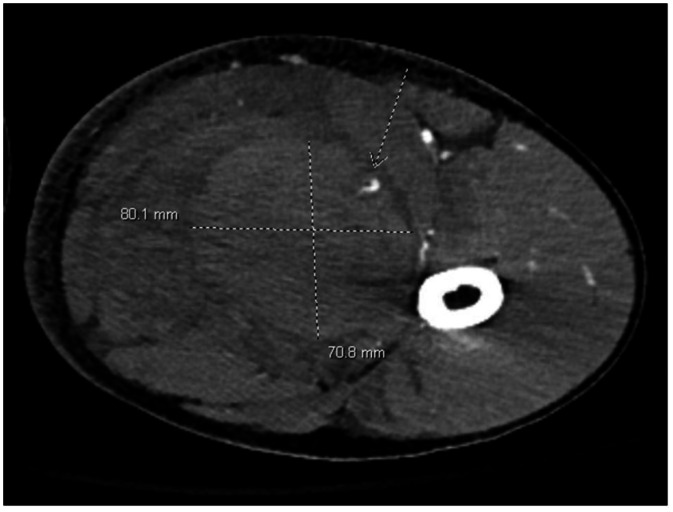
Axial contrast-enhanced CT image of the left thigh demonstrates a large intramuscular hematoma within the adductor compartment. A focal enhancing pseudoaneurysm is present centrally (dotted arrow), with associated compression of the adjacent femoral vein.
